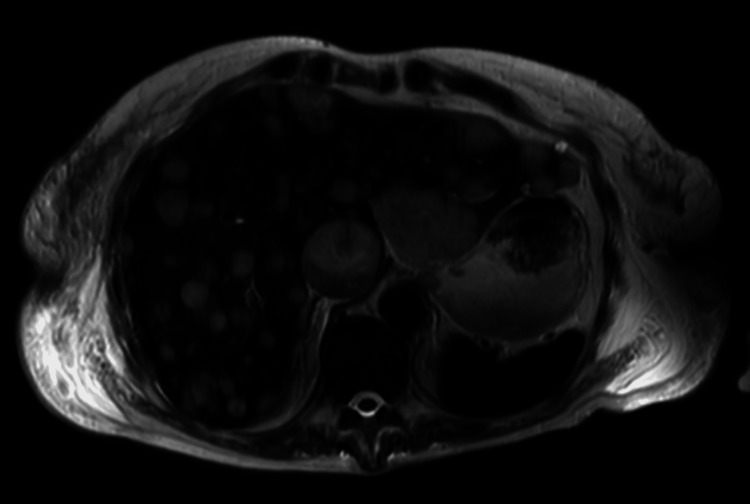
MRI of the liver MRI liver demonstrating multiple liver metastases.
